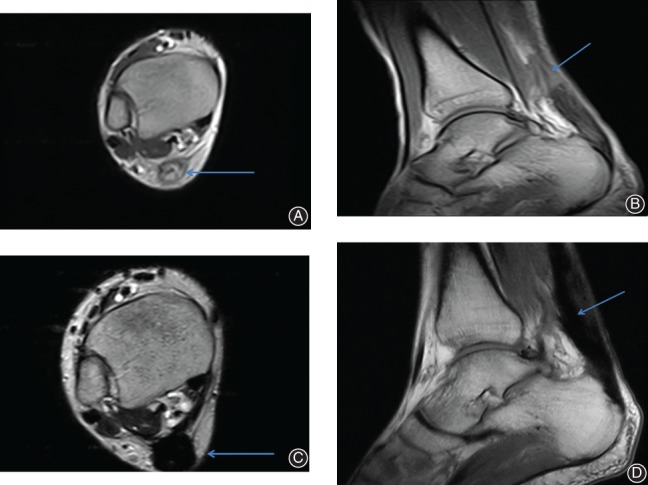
A 39‐year‐old man. The interval from rupture to surgery was 8 weeks. (A, B) MRI showed chronic Achilles tendon rupture in transaxial and sagittal planes (arrow). (C, D) MRI showed the continuity of the Achilles tendon with no signs of cysts or inflammation in transaxial and sagittal planes at the latest follow‐up (arrow).
